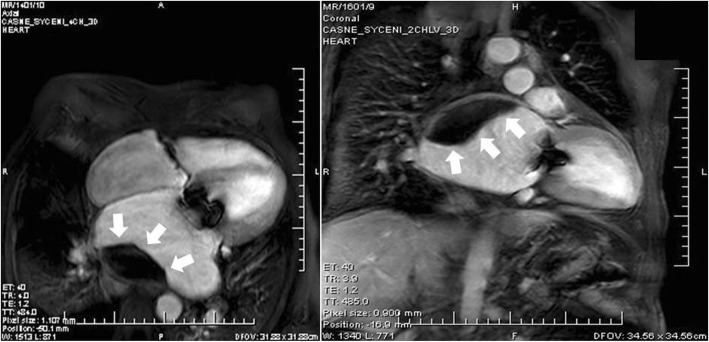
CMRI 3 months post-surgery CMRI – early gadolinium images, an apical four-(left figure) and two-(right figure) chamber view showing extreme dilatation of the LA with a large sessile thrombus (white arrows)
